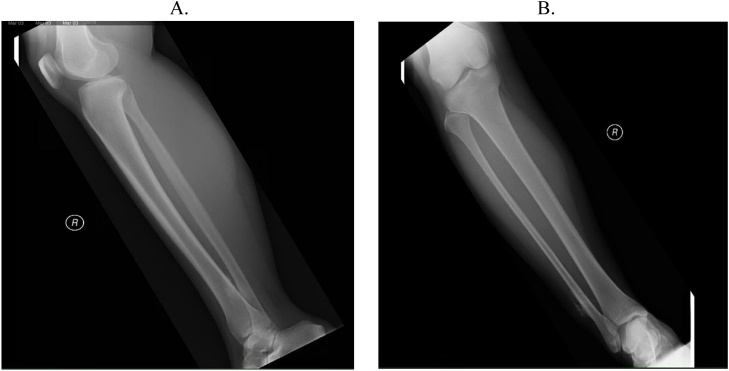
(A and B) Initial radiographs on presentation showing a soft tissue mass adjacent to the distal fibula vs. juxta cortical mass with punctate calcification centrally. Differential diagnosis radiographically included juxta cortical chondroma and parosteal osteosarcoma.
